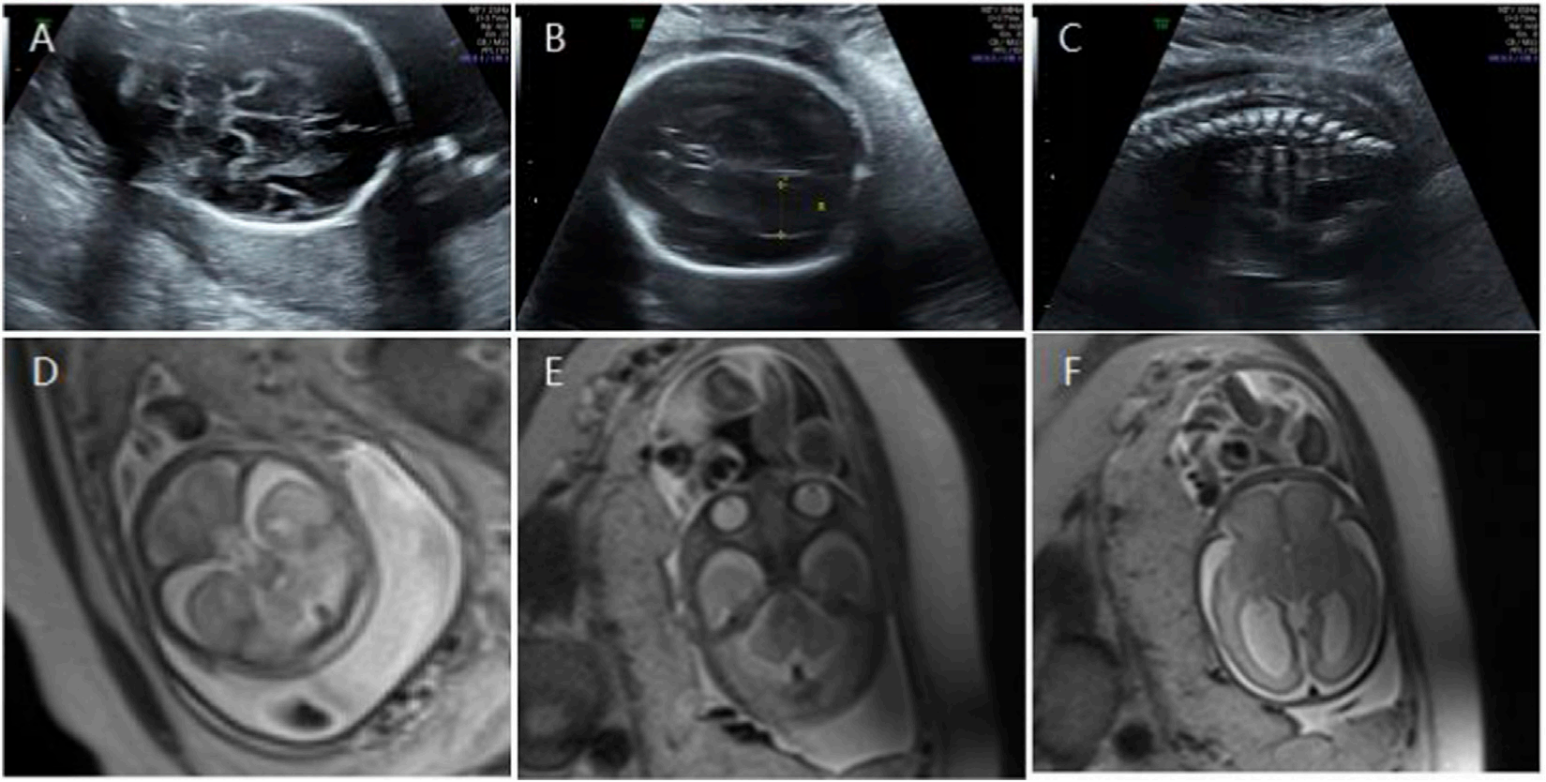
(A) Transverse ultrasound section showing the fetal cerebellum; transverse cerebellar diameter measured 23.4 mm (<–2.6 SD for gestational age). (B) Axial ultrasound view in the transventricular plane demonstrating bilateral ventriculomegaly, with atrial widths of 10.0 mm (left) and 13.6 mm (right). (C) Sagittal ultrasound view showing an abnormally positioned conus medullaris. (D,E) Axial T2-weighted fetal MRI confirming reduced cerebellar size. (F) Axial T2-weighted fetal MRI showing bilateral ventriculomegaly.
